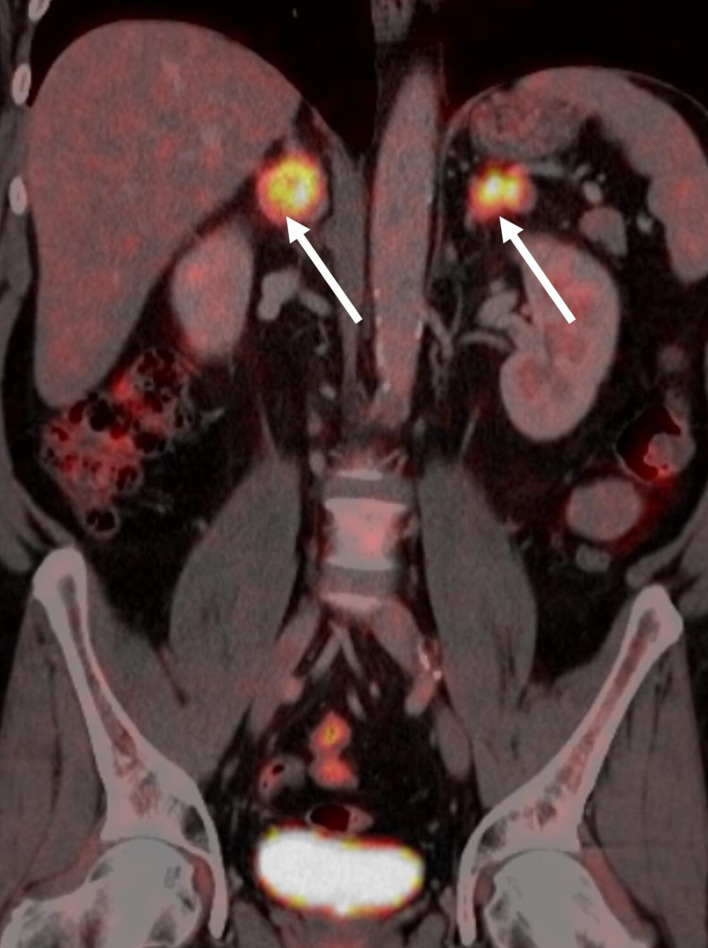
Coronal FDG PET/CT scan showing metabolically active, bilateral adrenal gland metastasis (arrow). FDG, fludeoxyglucose;PET, positron emission tomography.
